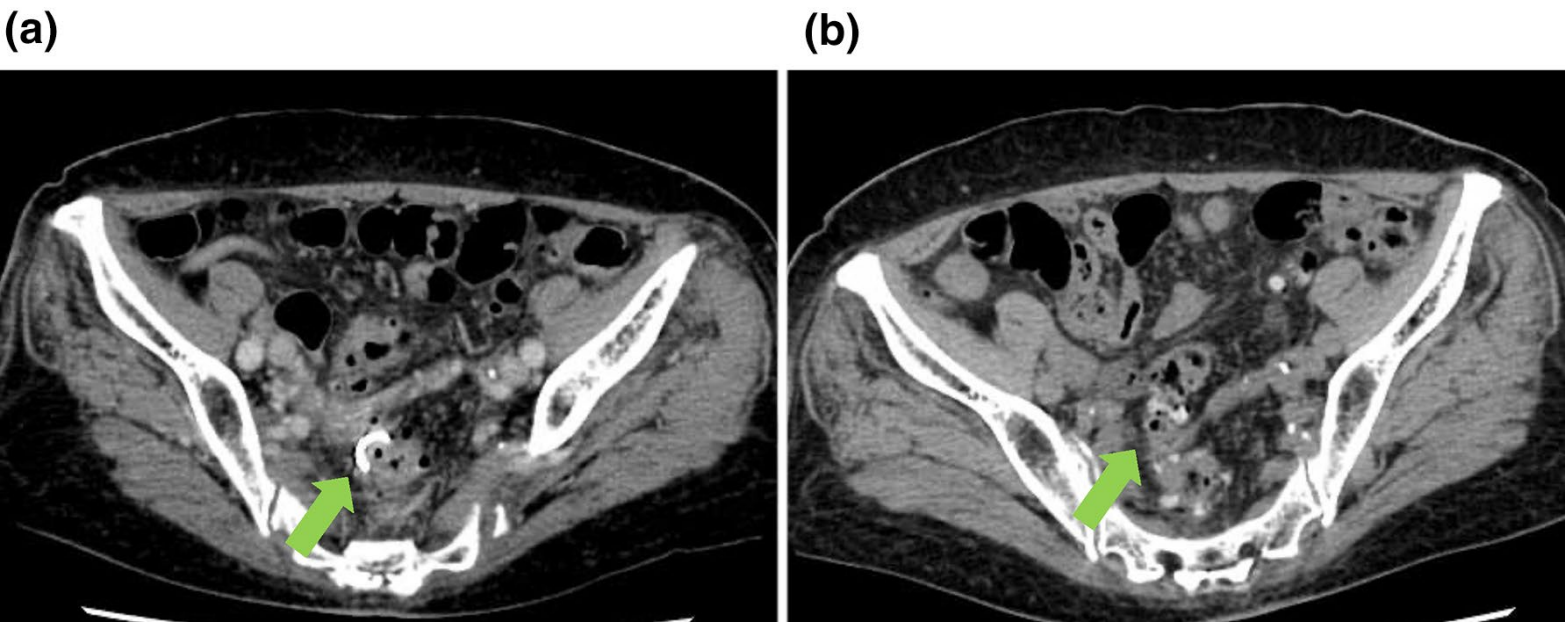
CT image of the abdomen a On the eighth day of hospitalization, with remains of the OTSC (green arrow) and no extraintestinal gas b One month after discharge, spontaneous detachment of the OTSC (green arrow)
